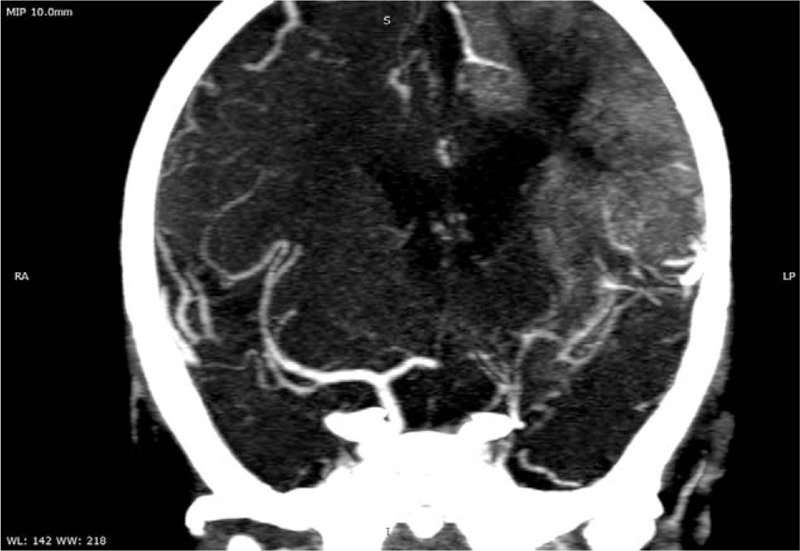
Angio CT, coronal section.
